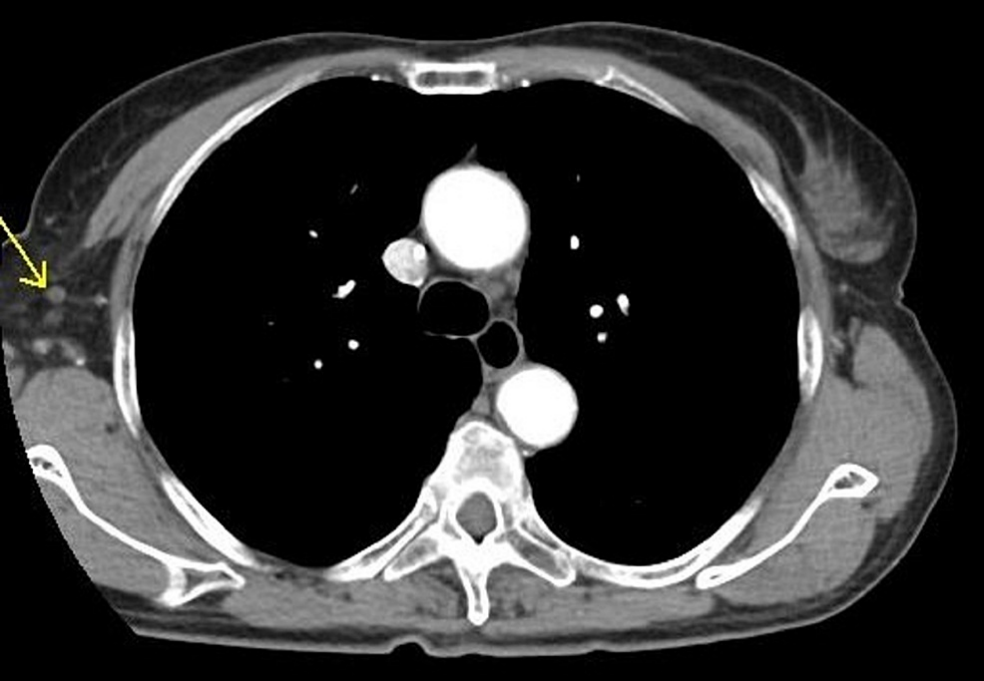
CT chest soft tissue window showing resolution of right axillary lymphadenopathy on 10/26/2018
